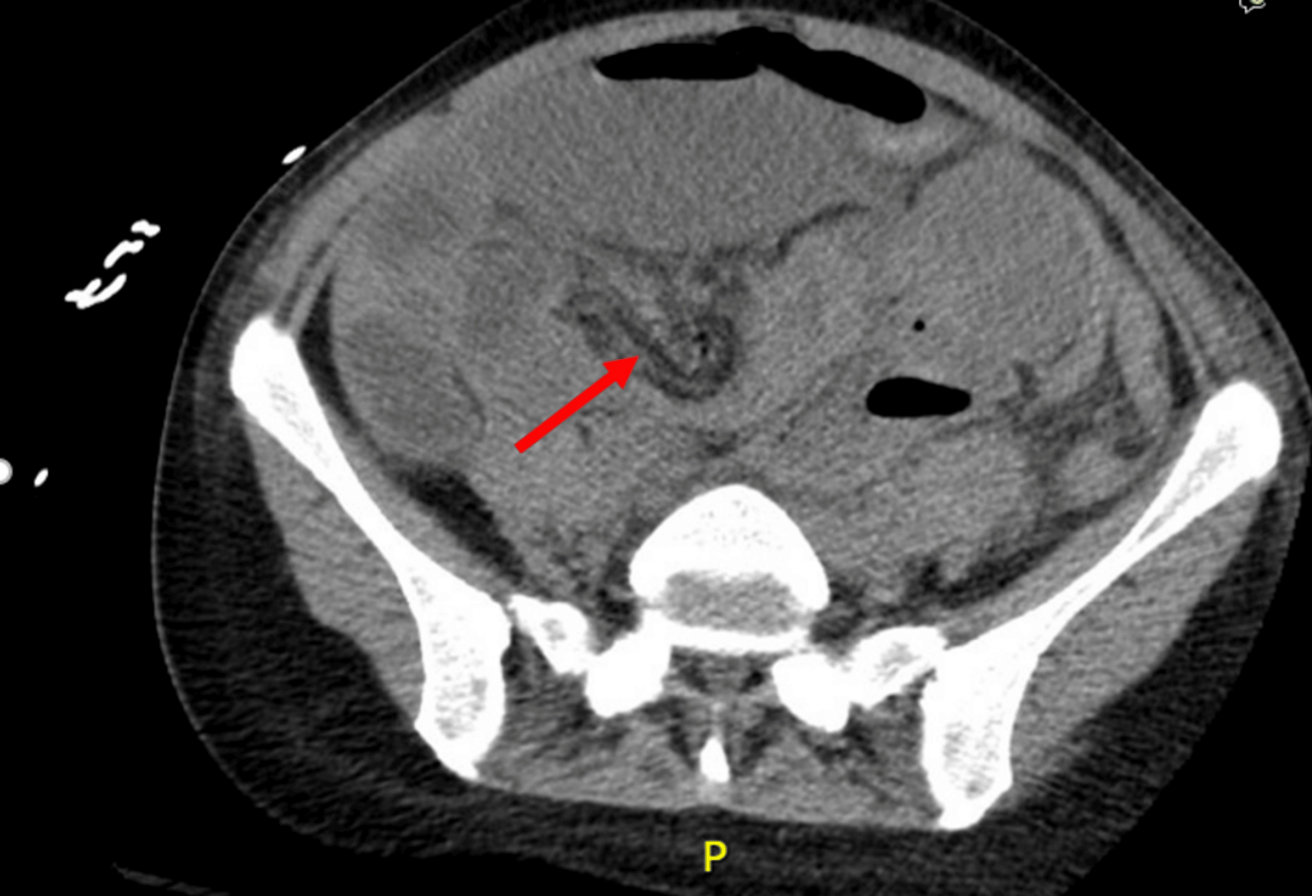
CT abdomen and pelvis without IV contrast (axial) Axial view of the CT abdomen and pelvis revealing swirling and edema of the mesentery (arrow). Also seen is dilation of the small bowel, which is notably seen in the right abdomen of this axial image.
